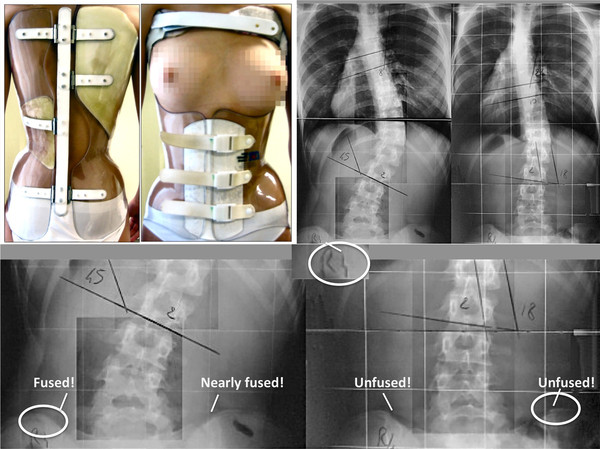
A sample of figures demonstrating that also in bracing it is not uncommon to treat mature individuals. The female patient on the upper left clearly is mature (Breast staging: Tanner 5). The x-rays as published within the same figure together with the clinical picture [26] on the first glance seem to show a drastical improvement of the curve as claimed by the authors. First of all, a permanent result like this cannot be achieved in a mature patient and is therefore not credible (on the left side x-ray a Risser 4 was estimated with fusion of the right iliac crest apophysis). Secondly, the Risser sign on the right x-ray seems more immature when compared to the left which could lead to the assumption that the right x-ray was the first and the left one was the last one and by this demonstrates a drastical curve progression. With kind permission of Pflaum, Munich (Weiss HR: Best practice in conservative scoliosis care. 4th edition in press).
